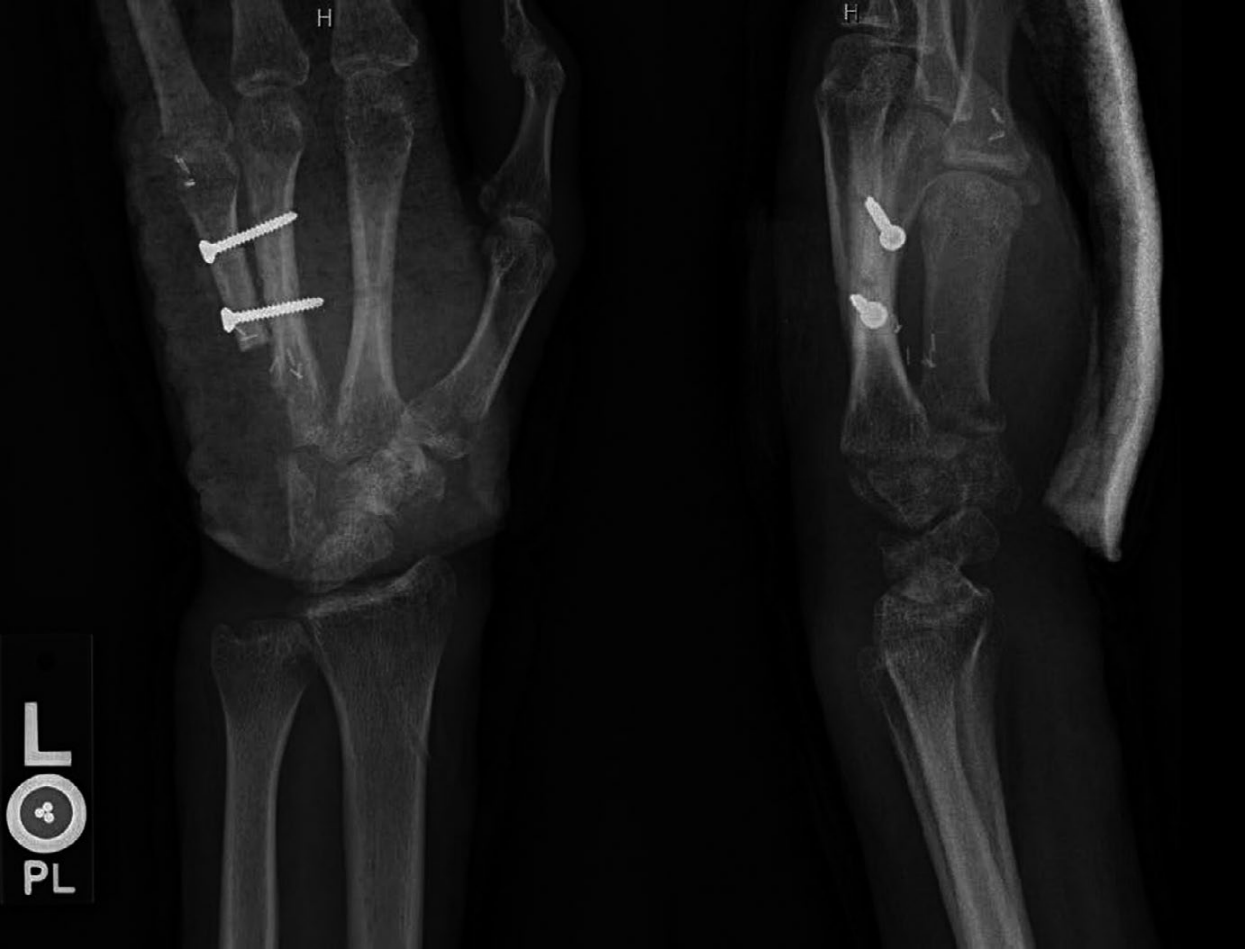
Intraoperative radiographs following removal of percutaneous pins and manipulation for improved range of motion
